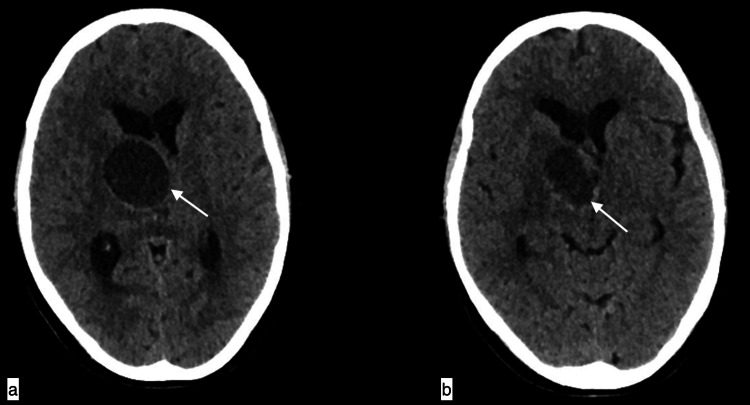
Intracranial thalamic hydatid cyst A 70-year-old female with an intracranial thalamic hydatid cyst presented with headache, left-sided weakness, and vomiting for three months. Axial CT brain showing a well-defined hypodense lesion of +16HU with hyperdense rim involving the right thalamus causing a mass effect in the form of midline shift towards left and compression of bilateral lateral ventricles and the foramen of Monro resulting in hydrocephalus.
